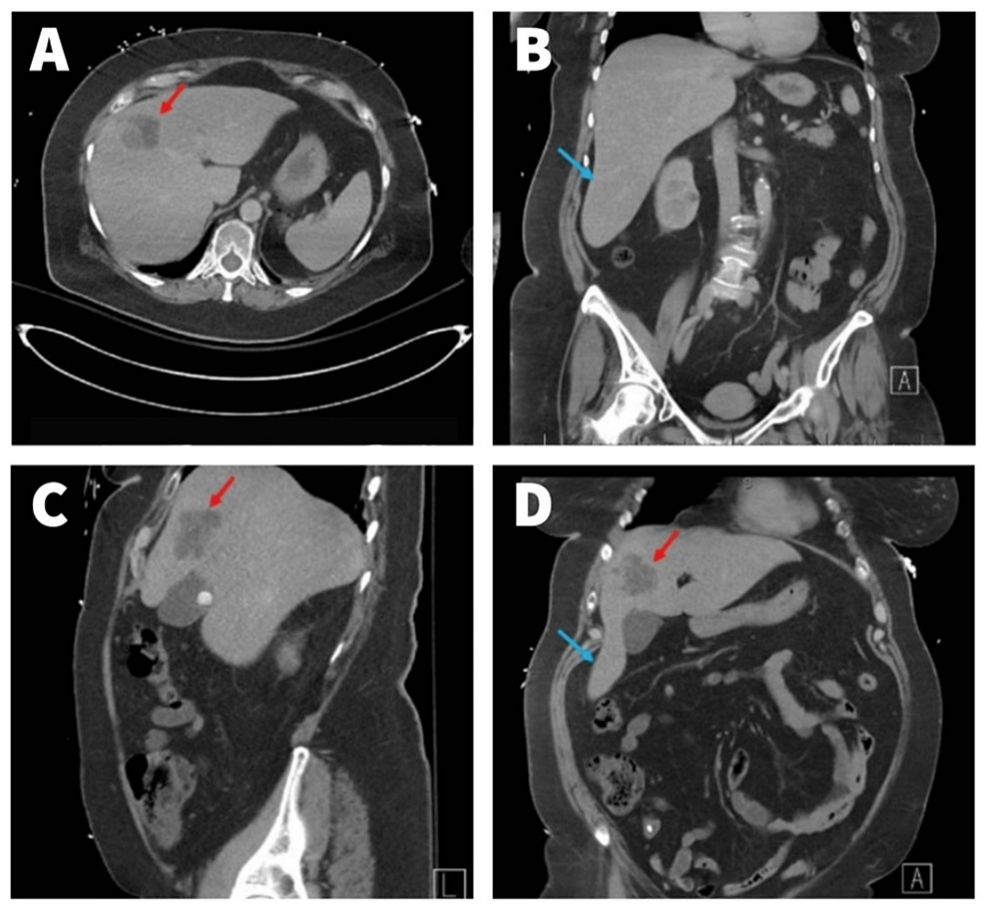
CT of the abdomen and pelvis. CT of the abdomen and pelvis showing lobulated mass within the left lobe of the liver (red arrows in A, C, and D) and tongue-like extension of the right lobe inferiorly (blue arrows in B and D). CT: computed tomography.
